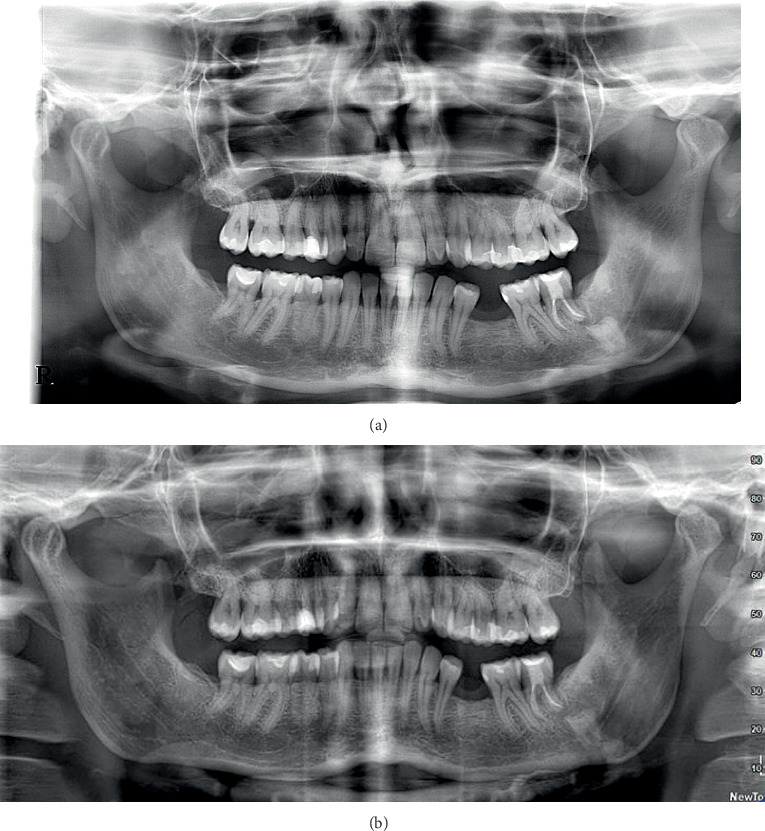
(a) Orthopantomography radiographic 2 years after coronectomy. (b) Orthopantomography radiographic 5 years after coronectomy.
